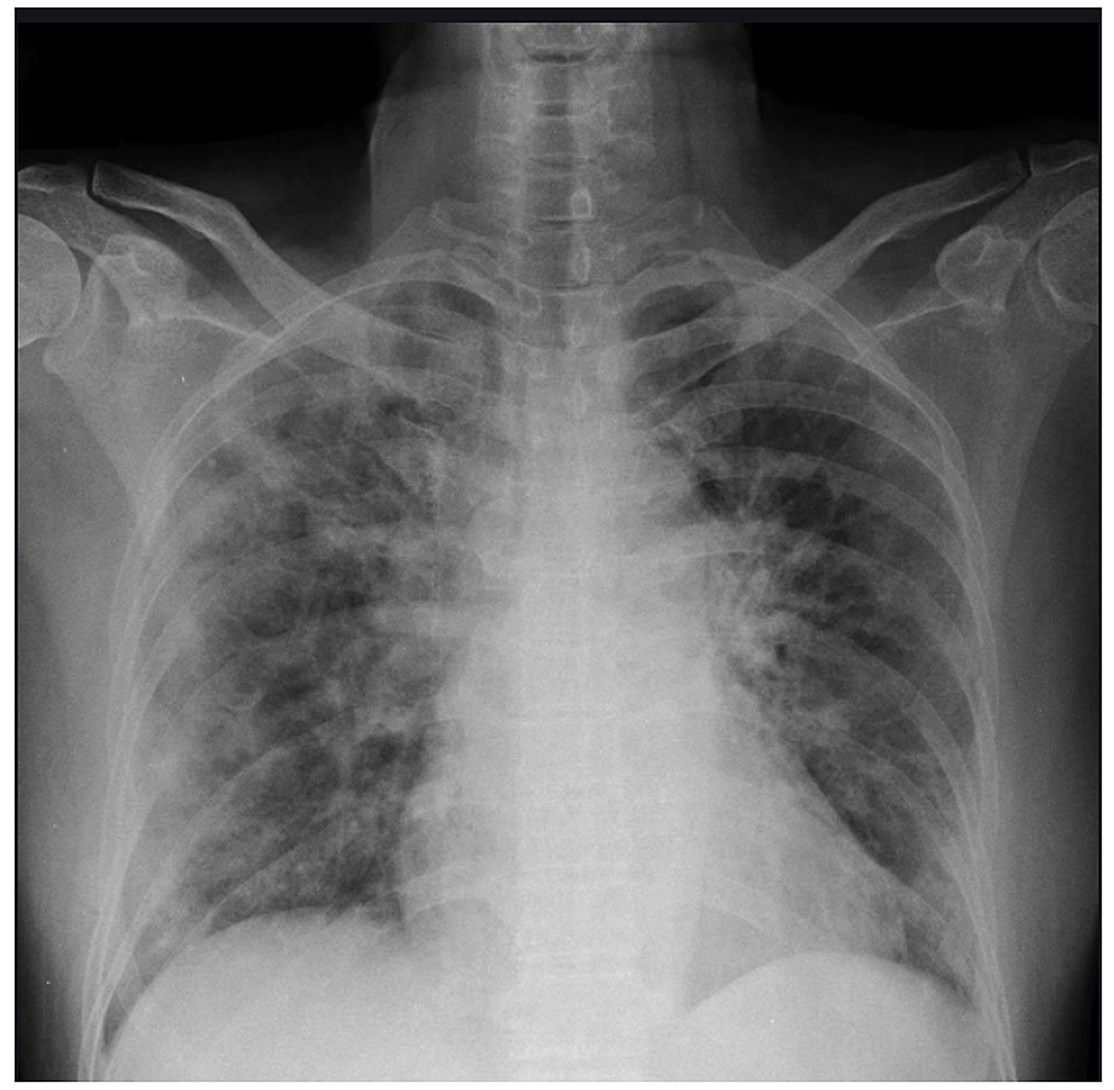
Chest X-ray showing bilateral ground glass opacities, typical for COVID-19
